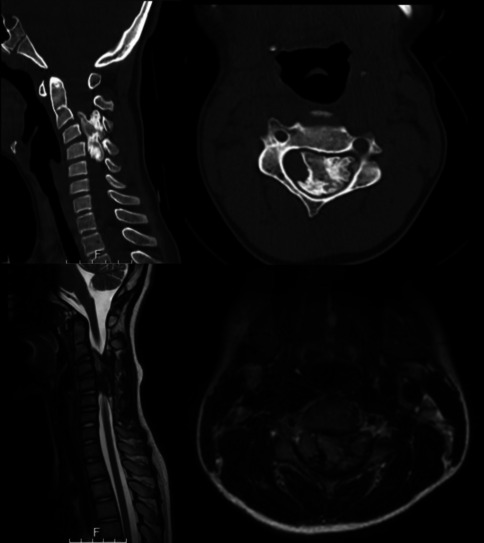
Preoperative non-contrast CT (above) and T2 MRI of cervical spine (sagittal and axial views) displaying a left eccentric calcified mass from C2 to C5 with severe spinal cord compression
